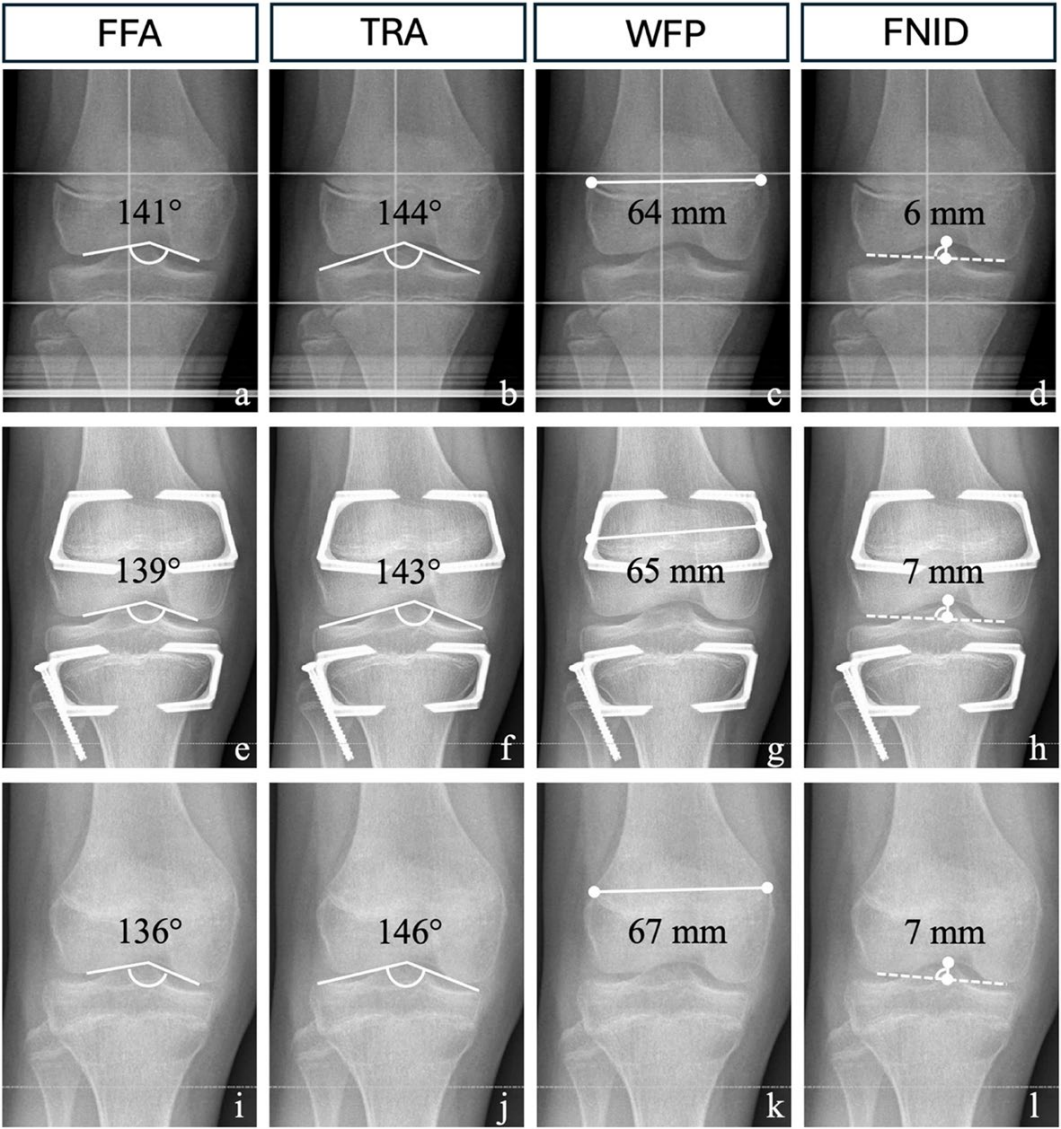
Intra-articular parameters before and after temporary epiphysiodesis. a-d Anteroposterior (a.p.) radiograph of the right knee joint of a 12-year-old girl with predicted idiopathic leg length discrepancy (LLD) of 2.8 cm. e-h a.p. radiograph of the right knee after 18 months of treatment by temporary epiphysiodesis around the knee using RigidTacks^TM^ (Merete, Berlin, Germany). i-l a.p. radiograph of the right knee 7 months after implant removal at last follow-up. a – Femoral floor angle (FFA). b Tibial roof angle (TRA). c Width at femoral physis (WFP). d Femoral notch-intercondylar distance (FNID). e FFA. f TRA. g WFP. h FNID. i FFA. j TRA. k WFP. l FNID
